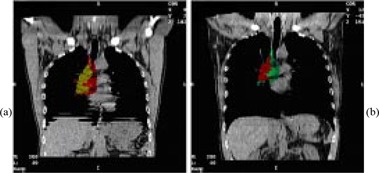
(a) Coronal slice reconstruction for free breathing during image acquisition (phase I). Gross tumor volume (GTV), yellow; PTV, red. (b) Coronal slice reconstruction for DIBH maneuver during image acquisition (phase II). GTV, red; PTV, green. (CT studies for two phases were performed at different times. That is why the colors chosen for outlining the target are not similar.)
